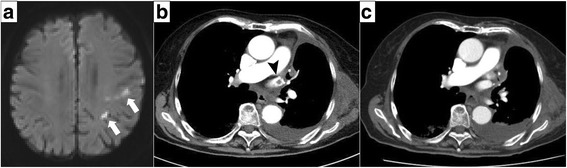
a Diffusion-weighted magnetic resonance image (MRI) of the brain showing multiple high-intensity signals in the area of the left middle cerebral artery (*arrow*). b Contrast-enhanced chest CT after the first episode of cerebral infarction showing a thrombus in the *left* superior pulmonary vein stump measuring 10 mm in diameter (*arrow head*). c Thrombus in the left superior pulmonary vein stump disappeared on follow-up contrast-enhanced chest CT scan after the second episode of cerebral infarction
